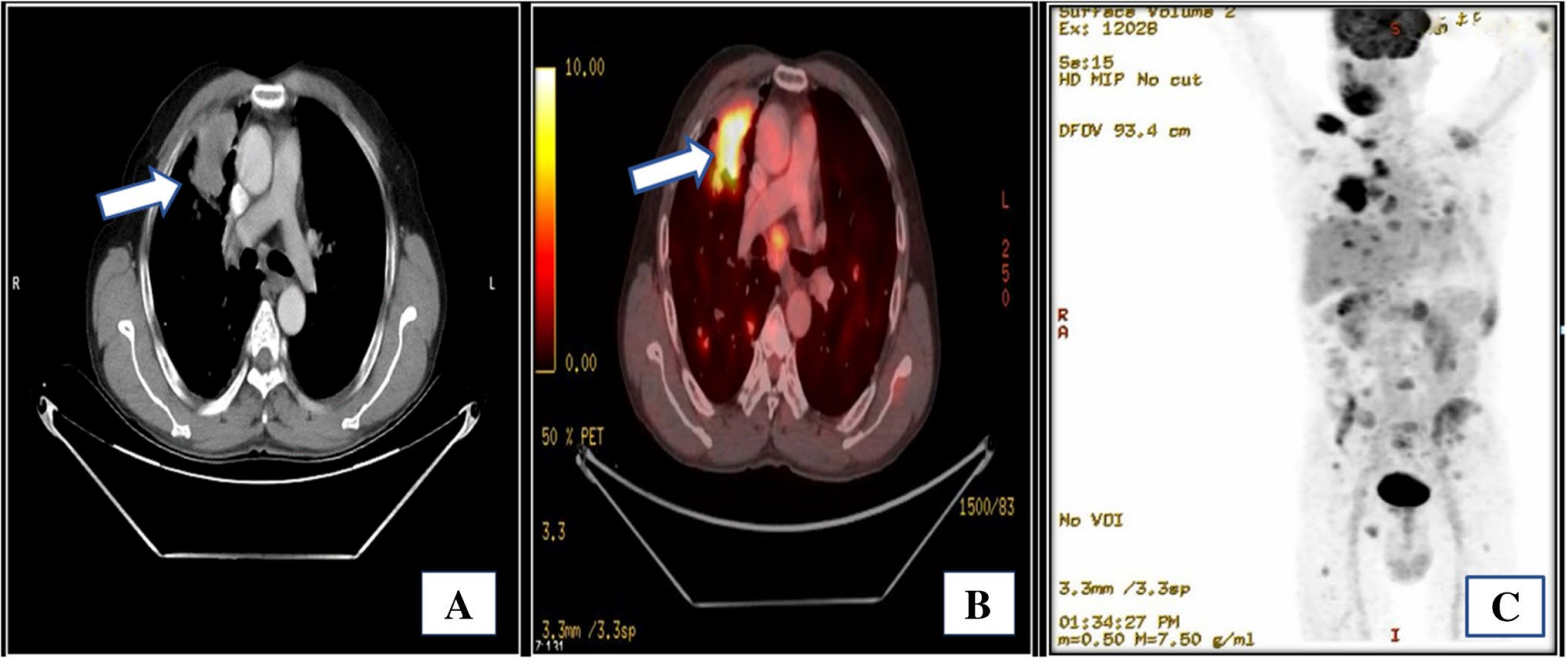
A and B F-18-FDG PET/CT (flurodeoxyglucose F18 positron emission tomography/computed tomography) scan shows FDG avid pleura-based mass in the upper lobe of right lung: ~ 68 × 48 × 64 mm, maximum standardized uptake value (SUV max) 10.9 (arrow). It abuts the right chest wall anteriorly; no obvious erosion noted. C Hypermetabolic metastatic lesions in bilateral lung nodule, liver, extensive sclerotic, and few lytic skeletal lesions and bilateral adrenal
